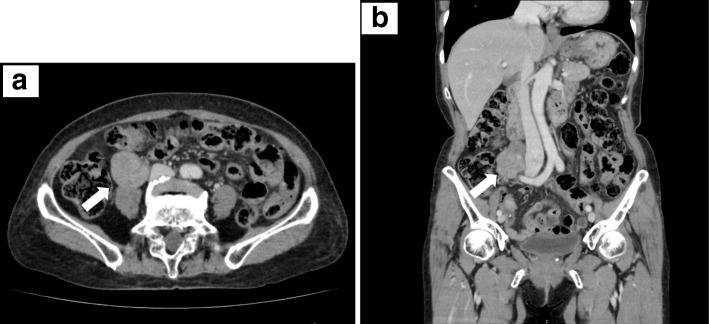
Contrast-enhanced computed tomography of the leiomyosarcoma. Axial (a) and coronal (b) contrast-enhanced computed tomography of the patient’s abdomen revealing a well-defined, lobulated solid mass (white arrows) with a diameter of 5.5 cm in the right retroperitoneal space
